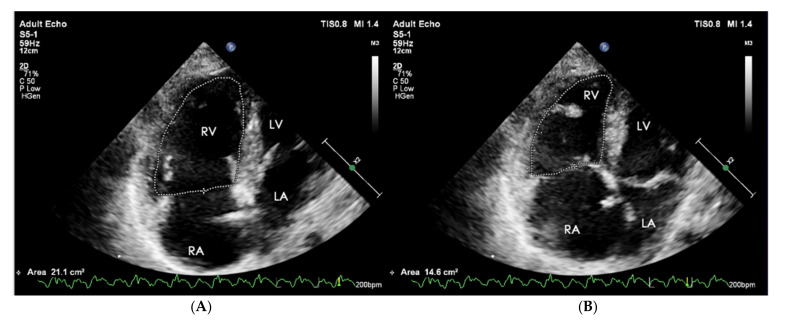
(A) End-diastolic volume RV = 21.1 cm^2^, (B) end-systolic volume RV = 14.6 cm^2^. FAC = 30.8%. FAC = A(diast) − A(sys)/A(dias) * 100%. 2D-TTE, 4CH view. RV: right ventricle; FAC: functional area change; 2D-TTE: two-dimensional transthoracic echocardiography; 4CH: four-chamber.
